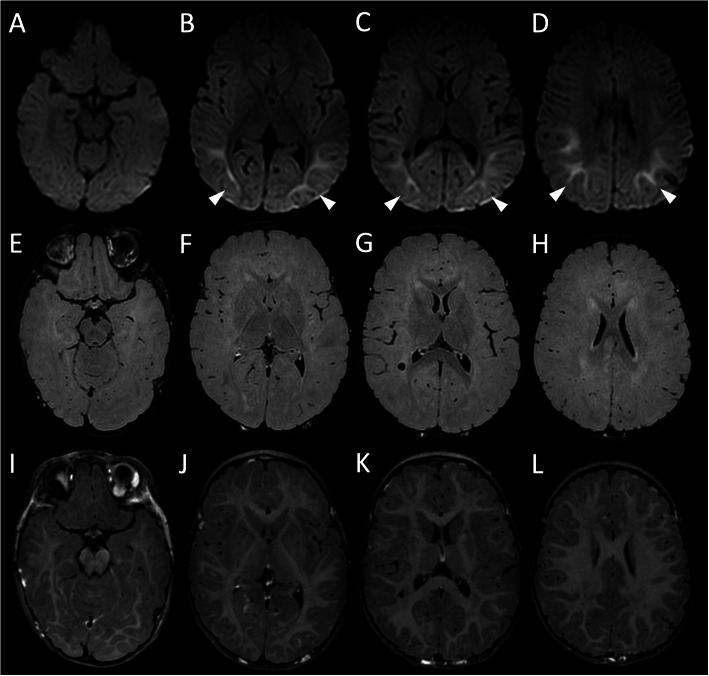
Brain MRI performed at clinical onset. a-d Axial diffusionweightedimages reveal restricted diffusion in the cortico-subcortical parieto-temporal regions (arrowhead), likely secondary to seizure-related changes. Axial contrast-enhanced FLAIR (e-h) and T1-weighted (i-j) images reveal normal ventricular size and absence of leptomeningeal contrast enhancement
